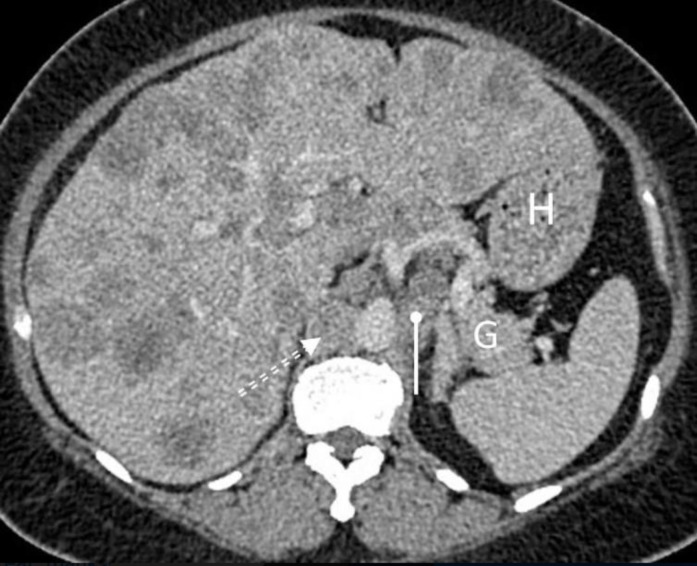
Contrast enhanced axial CT abdomen of a 56-year-old female with a history of carcinoma of the breast shows multiple, irregular low-density areas with heterogenous enhancement in the liver, suggesting metastasis. Note the enlarged retrocrural (double dashed arrow) and paraaortic (oval arrow) lymph nodes. Distal portion of body and tail of the pancreas (G) is seen adjacent to the splenic hilum. Stomach (H) is filled with food particles
